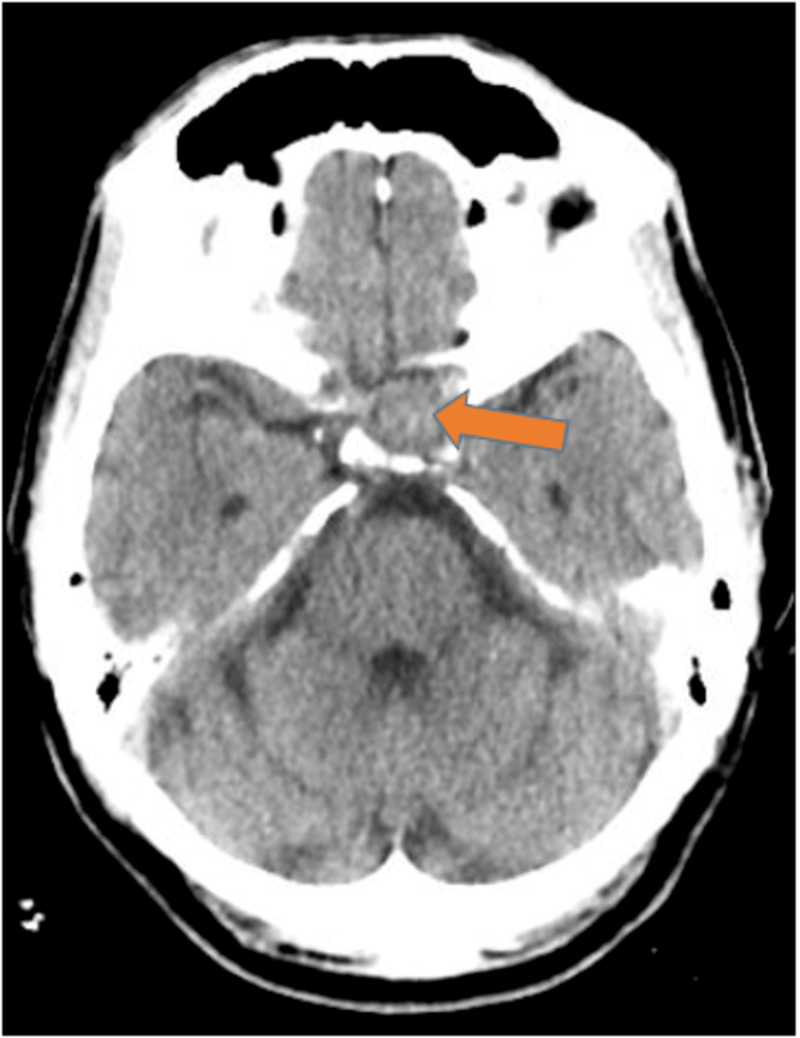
CT head with IV contrast showed a large 2.2 x 1.9 cm mass in the region of the pituitary gland splaying the vascular system as shown by the orange arrow.
